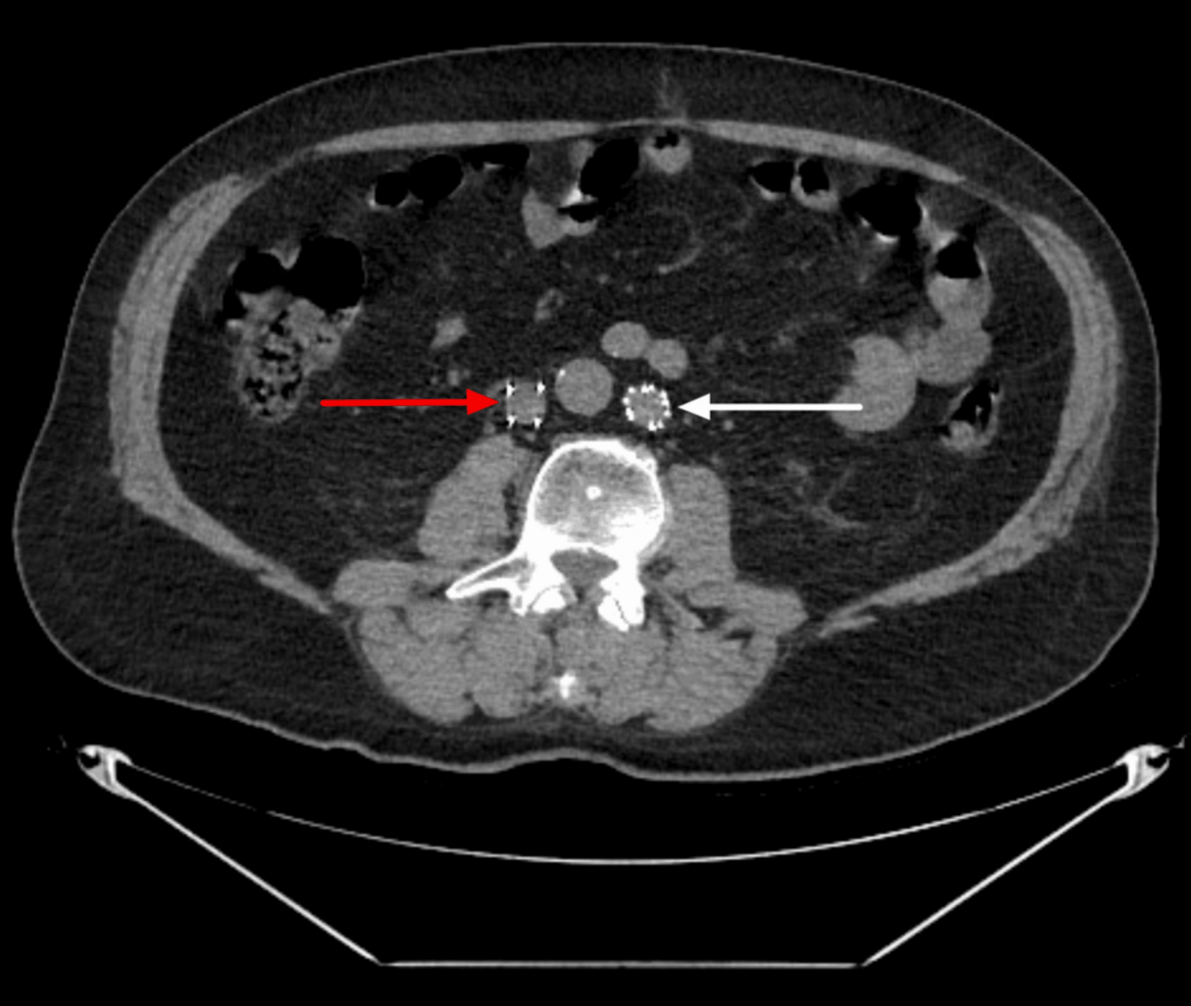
Axial CT demonstrates a filter in the right IVC (red arrow) and the left IVC (white arrow). CT, Computed tomography; IVC, Inferior vena cava
